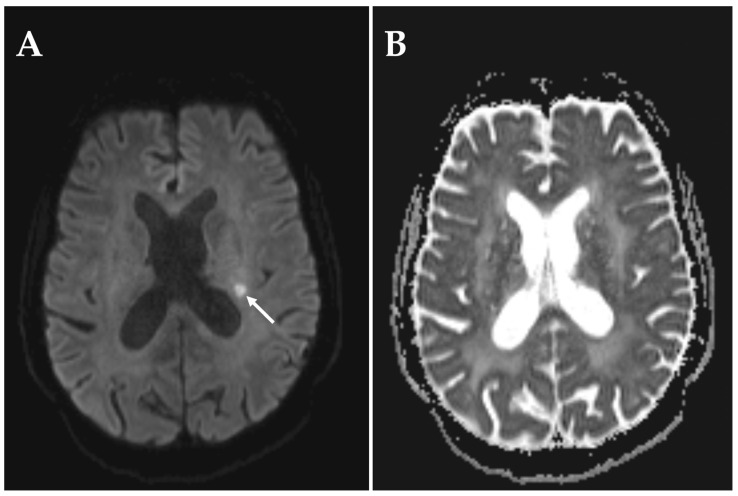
MRI sequences revealing subacute infarction in a patient with NAION of the right eye. MRI was performed on a 3 T scanner two days after onset of visual impairment. (A) DWI showing a hyperintensity in the subcortical territory of the left middle cerebral artery. (B) The corresponding region in the ADC shows normal signal behavior.
